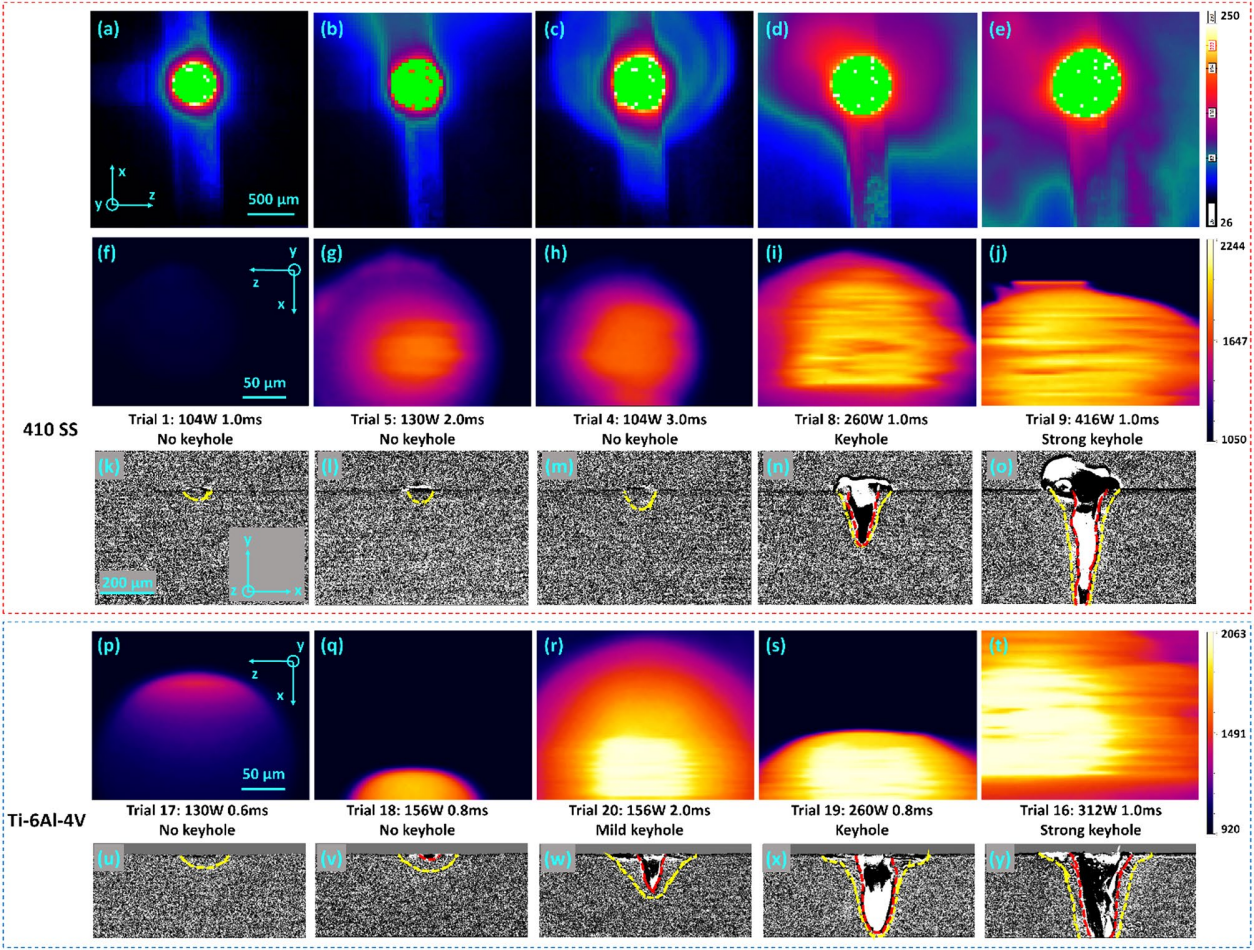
(a–e) The high-speed IR observations of different processing conditions at the frame right before the laser shuts off. The last two (d and e) have strong vapor plumes indicating a keyhole. (f–j and p–t) The high-spatial IR observations of different processing conditions at the frame right before the laser shuts off where i, j, r, s, t have fringe patterns, which indicate a keyhole. (k–o and u–y) The corresponding x-ray images right before the laser shuts off, where yellow dashes indicate the solid–liquid boundary and red dashes indicate the liquid–gas boundary. (Temperatures all in °C).
